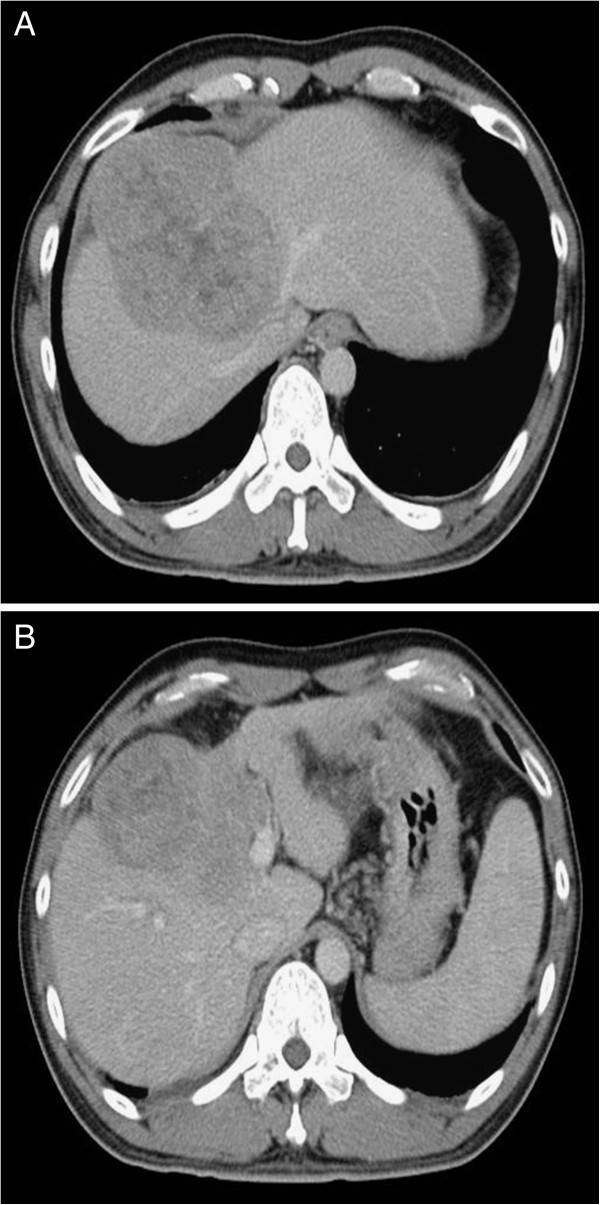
Computed tomography (CT) scan images before surgery. (A) Large liver tumor with diaphragmatic invasion and occlusion of the middle hepatic vein. (B) Large liver tumor with tumor thrombus in the left portal vein.
